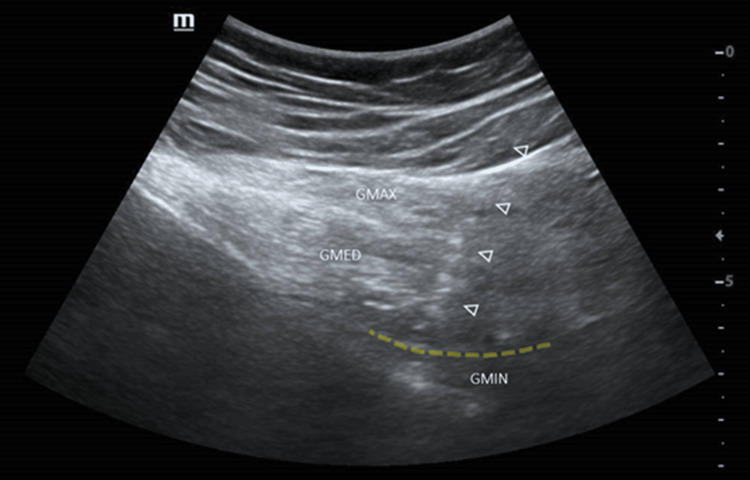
Ultrasound image demonstrating the infiltration of MSCs into the fascia between the gluteus minimus and gluteus medius Arrowheads: needle; dotted yellow line: fascia between the GMIN and GMED GMIN: gluteus minimus; GMED: gluteus medius; GMAX: gluteus maximus
